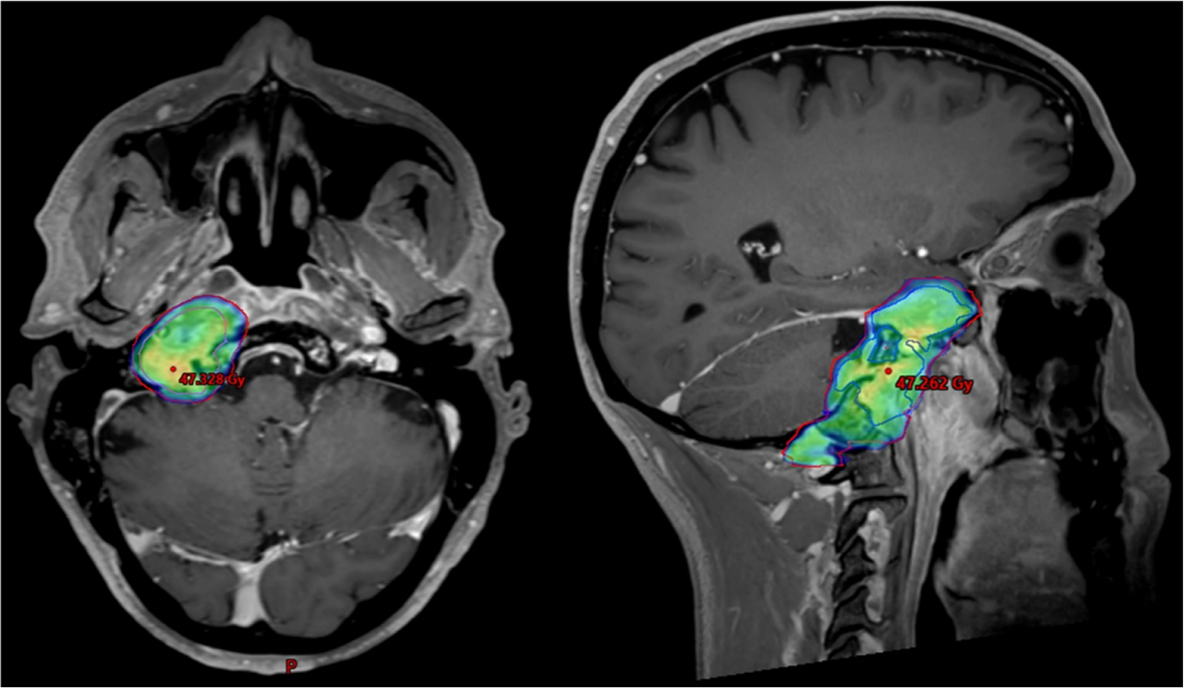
Treatment plan for stereotactic re-re-irradiation using rotational intensity-modulated radiotherapy (volumetric modulated arc therapy; VMAT). The 95%-isodose coverage of the prescribed 45 Gy is shown on fused MRI in axial and sagittal plane, with the latter demonstrating extensive craniocaudal (including prevertebral) tumor extension
